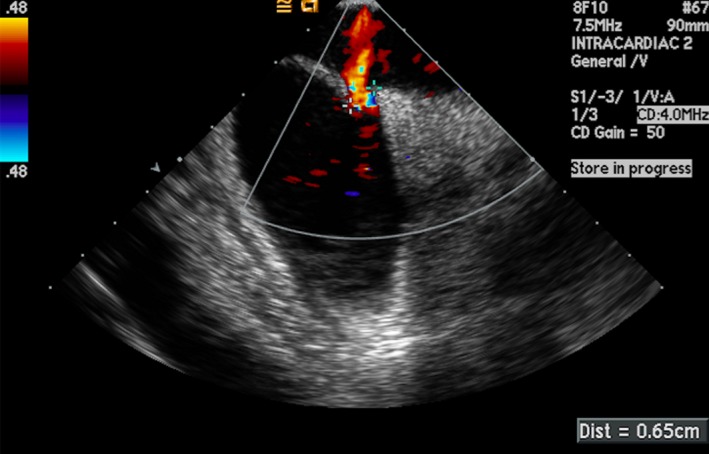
Intracardiac echocardiogram (ICE) image showing a PFO measuring 6.5 mm using color Doppler.
